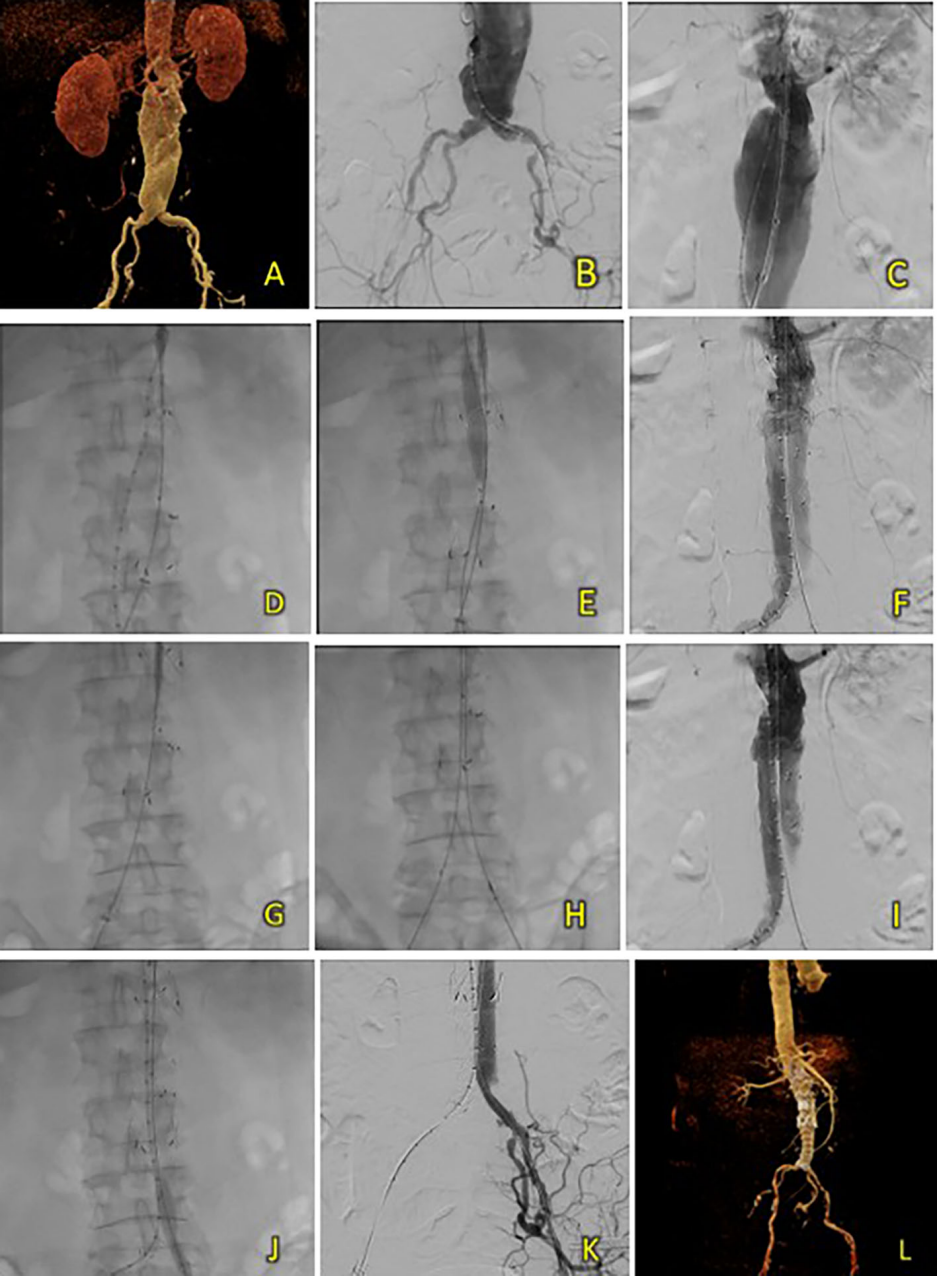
T&K B-EVAR on Patient 5. (A) Pre-procedure CT scan. (B-C) Procedural diagnostic angiography. (D-E) Deployment of main body stent. (F) Angiographic evaluation revealing the expansion of graft stent. (G-H) deployment and ballooning of extensions. (I) Angiographic evaluation with reduced flow through the left extension limb. (J) Ballooning of limb extension. (K) After the angiography evaluation after additional limb extension deployment, the results showed good flow and no endoleak. (L) Post-procedure CTA was showing promising results.
